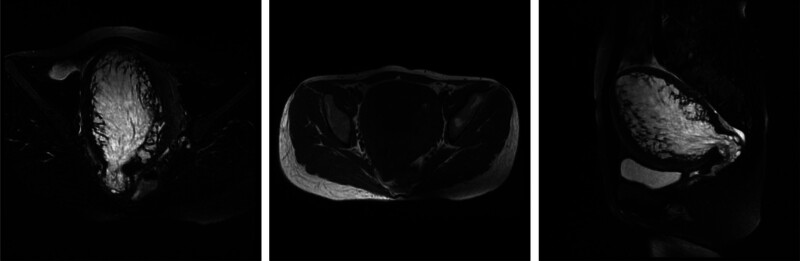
Contrast enhanced MRI of pelvic. MRI = magnetic resonance imaging.
